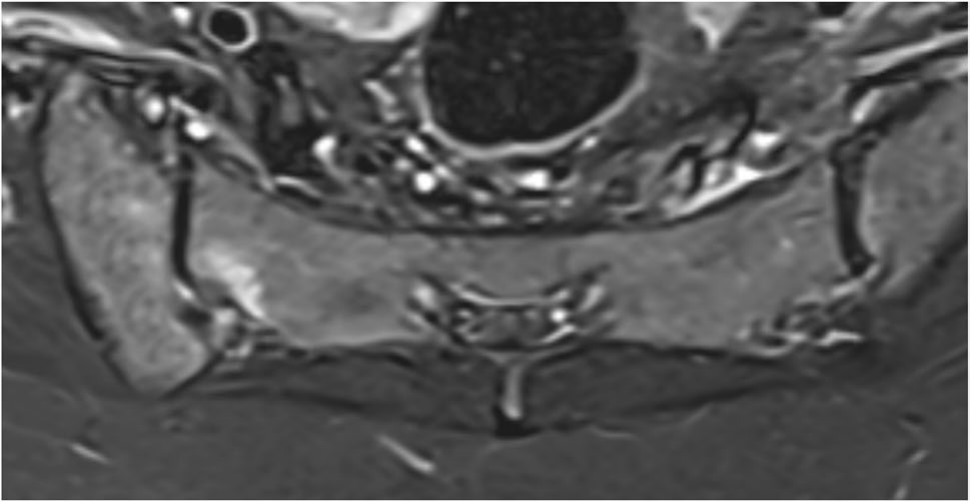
A 41-year-old woman with a right accessory SIJ showing edematous and sclerotic changes of the facing bones on axial oblique FS T2-weighted image
